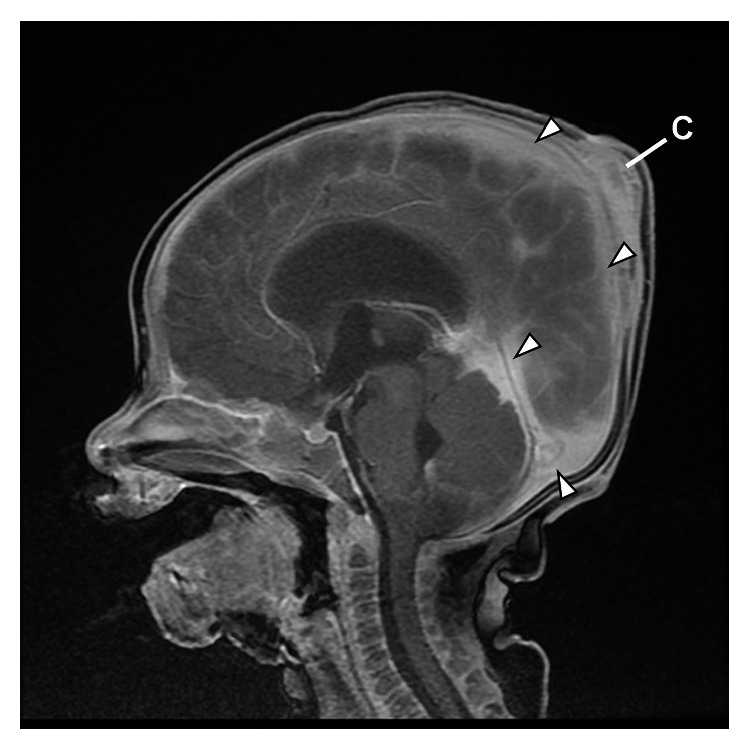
Hospital day 63: MRI of the head showing trace cerebral (falx and tentorium) and posterior fossa subdural collections (empyema) (arrows). C: cephalhematoma.
